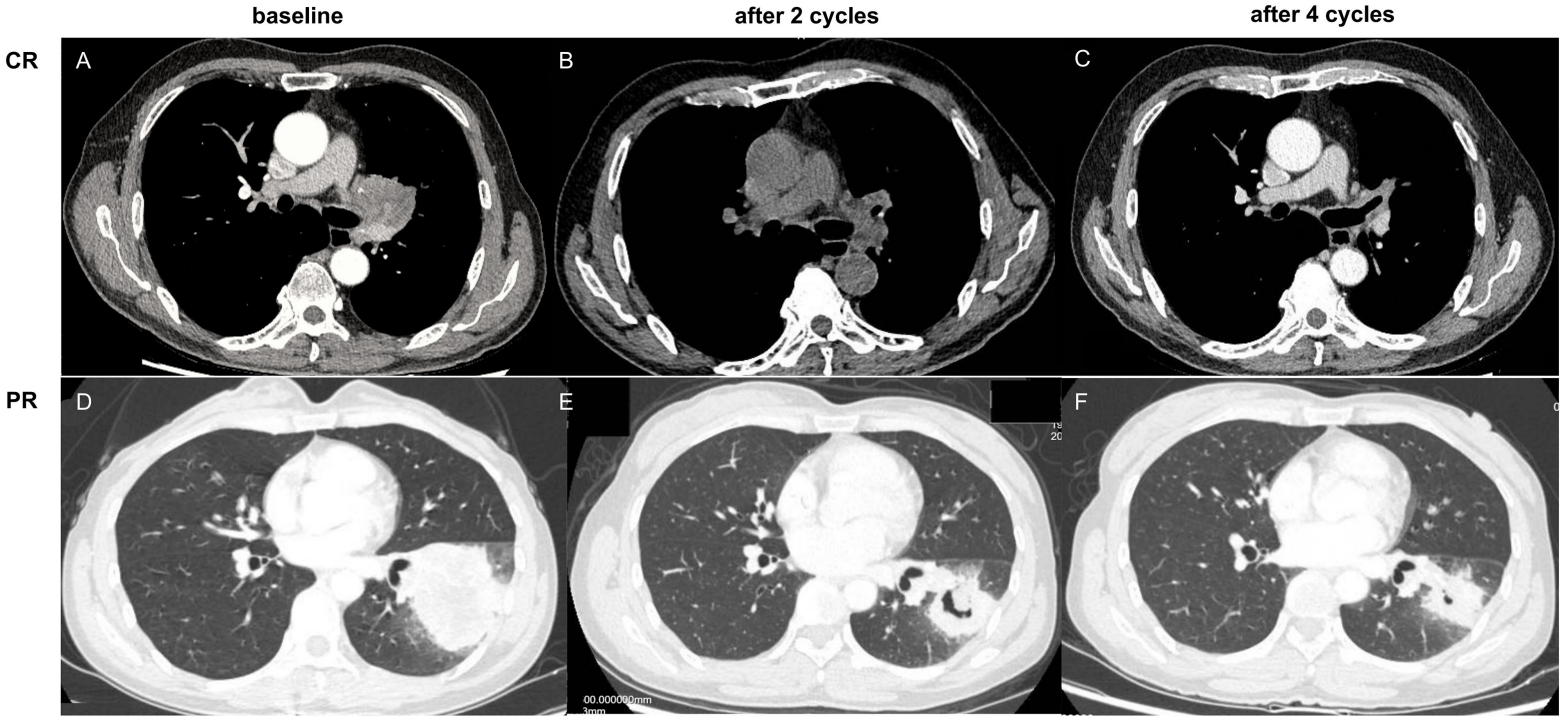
(A–C) complete response (CR) based on computed tomography, as notumor issue was found (0.0%); (D–F) partial response (PR) based on computed tomography, as tumor regression ≥30%.
